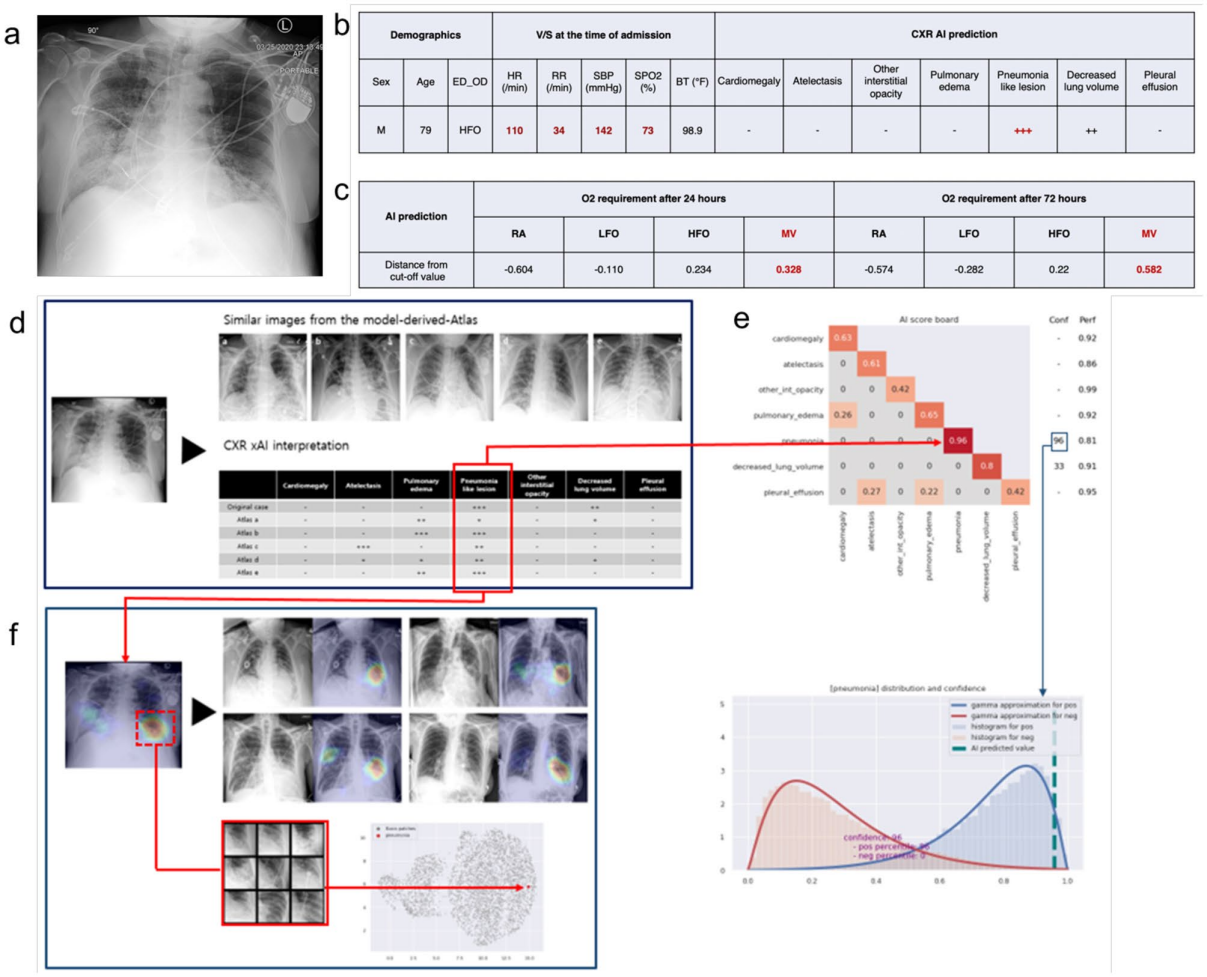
AP CXR of a 79-year-old man with COVID-19 and respiratory insufficiency and AI prediction of most likely stage of oxygen requirement at 24 and 72 h from the time of ED admission. (a) AP CXR obtained on ED admission, (b) Clinical information and infection-associated radiographic labels identified by our CXR xAI model, (c) Final prediction of oxygen requirement after 24 and 72 h. Our COVID-19 xAI model predicted that the patient would require MV after 24 and 72 h, (d) Prediction probabilities for 7 infection-associated radiographic labels are summarized in the interpretation table and 4 similar CXRs with characteristic findings for pneumonia were selected from the Model-Derived Atlas, (e) Pneumonia was identified as a significant feature, based on high prediction probability and calibrated classifier confidence on the AI scoreboard, (f) Pneumonia was correctly localized by Grad-CAM, and on UMAP, the test patch was closely located to corresponding patches from the Model-Derived Atlas in the embedding space after dimensionality reduction.
